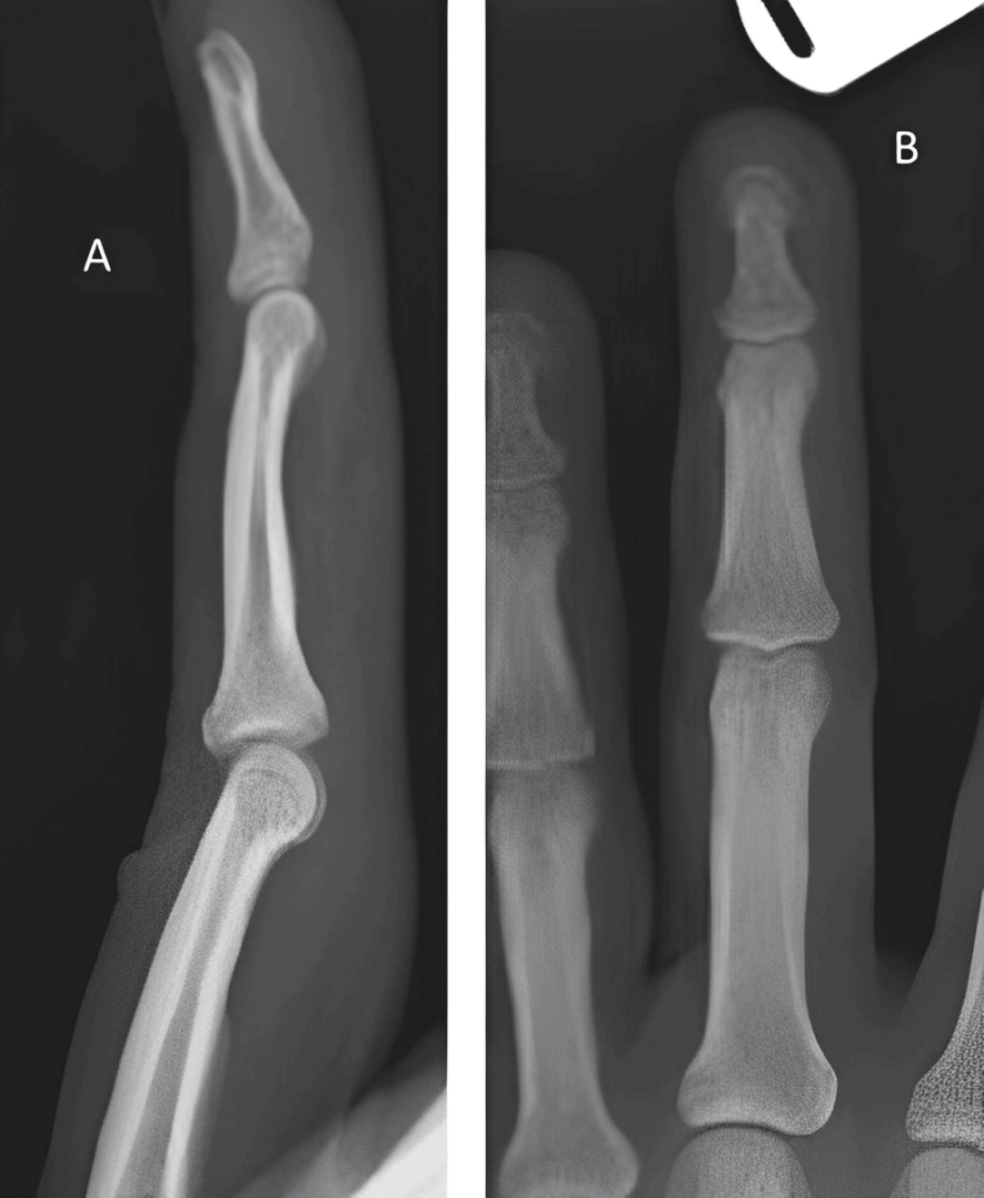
Normal radiograph of the middle finger (A) Sagittal view. (B) Posteroanterior view.
